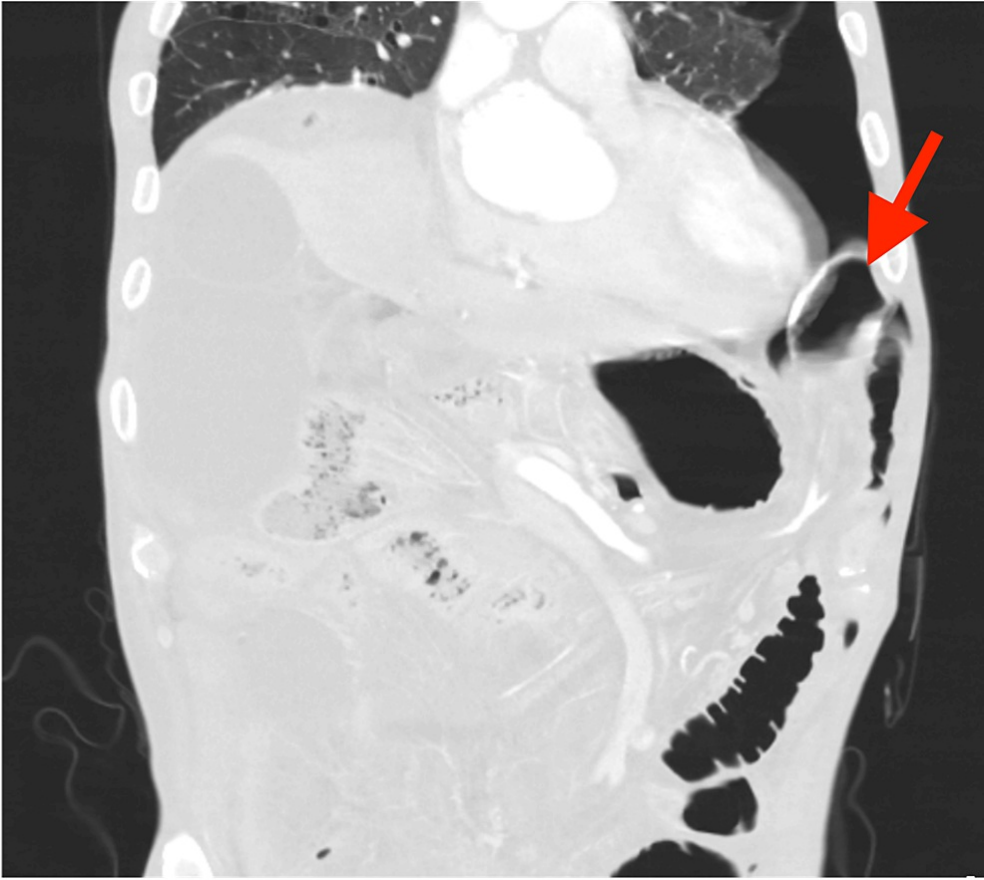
Chest computed tomography showing pneumothorax and diaphragmatic hernia
